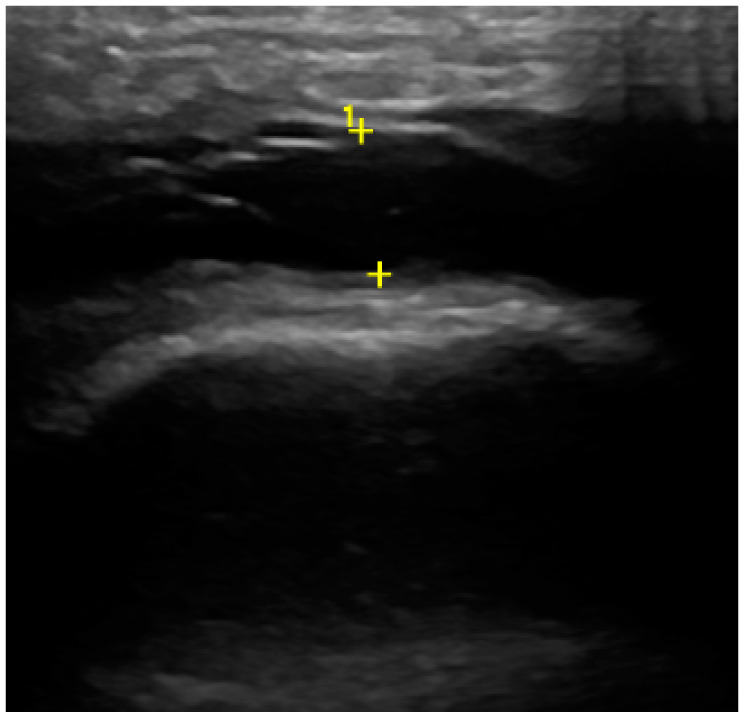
Ultrasound (effusion within the joint).
